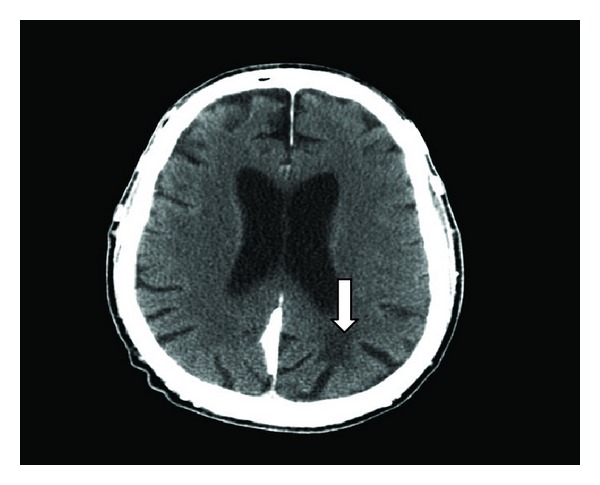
Nonenhanced CT scan shows a hypodensity in the left parietal lobe.
